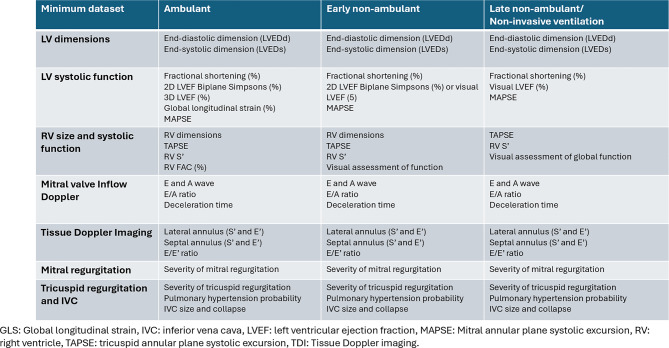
Minimum echocardiography dataset for ambulant, early non-ambulant and late non-ambulant/non-invasive ventilation stages
